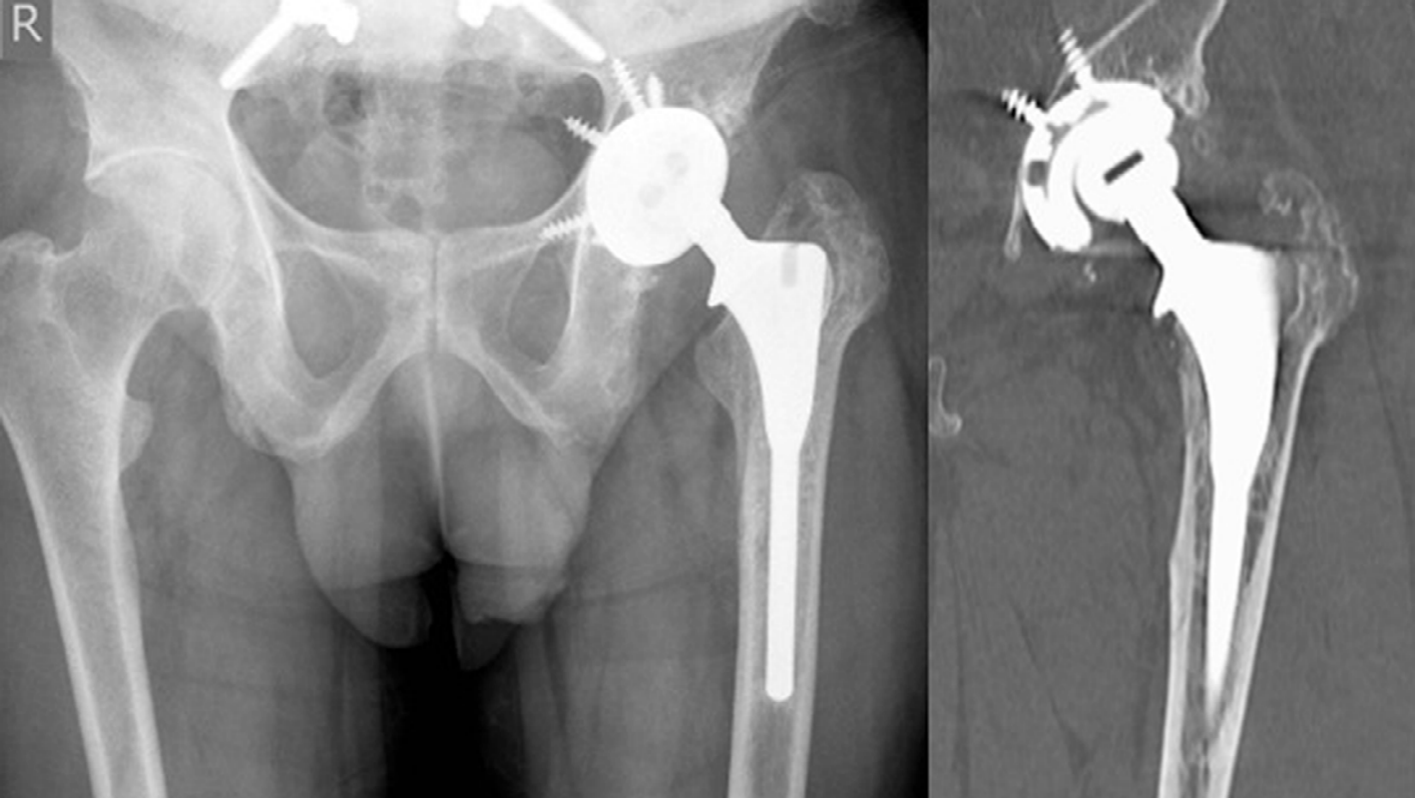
Plain radiograph shows an undisplaced proximal femur periprosthetic fracture involving lesser trochanter. CT shows eccentric wear of the articular surface.
